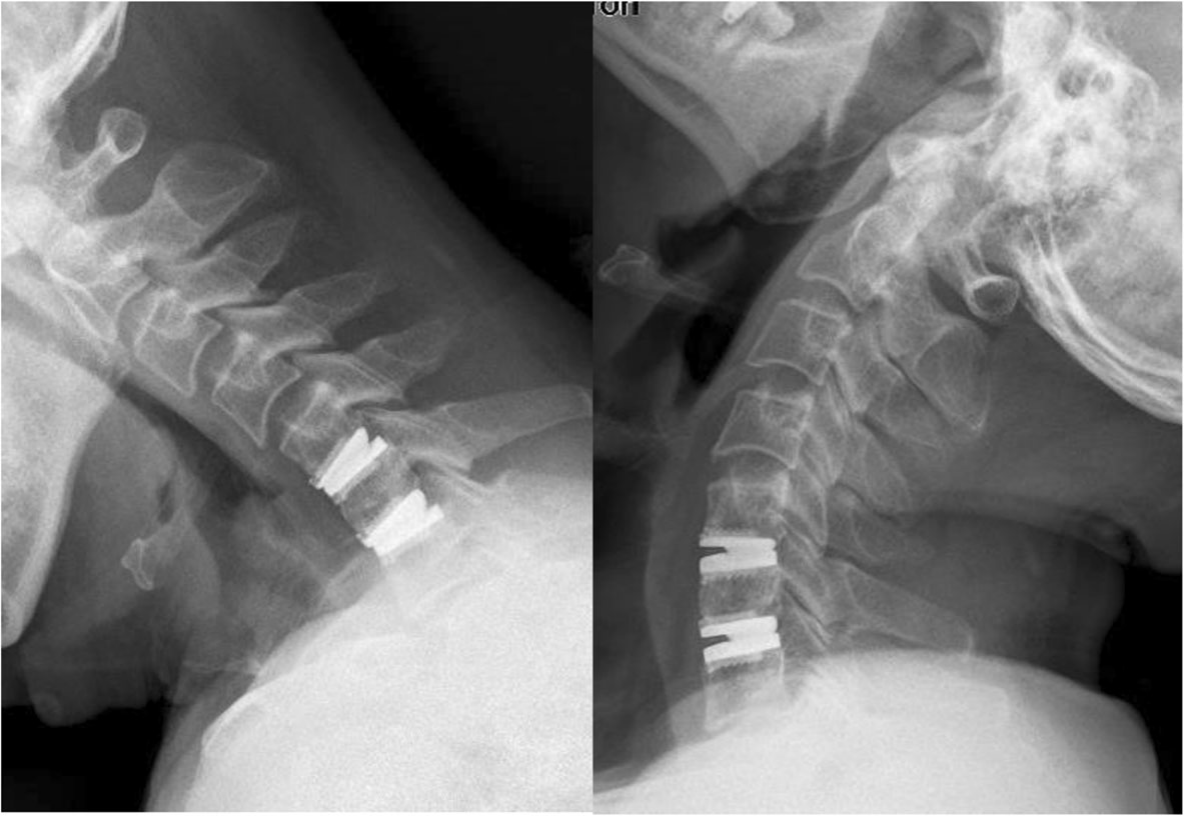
Lateral flexion and extension radiographs showing satisfactory prosthesis mobility at C5/6 and C6/ 7 at 89 months after surgery
